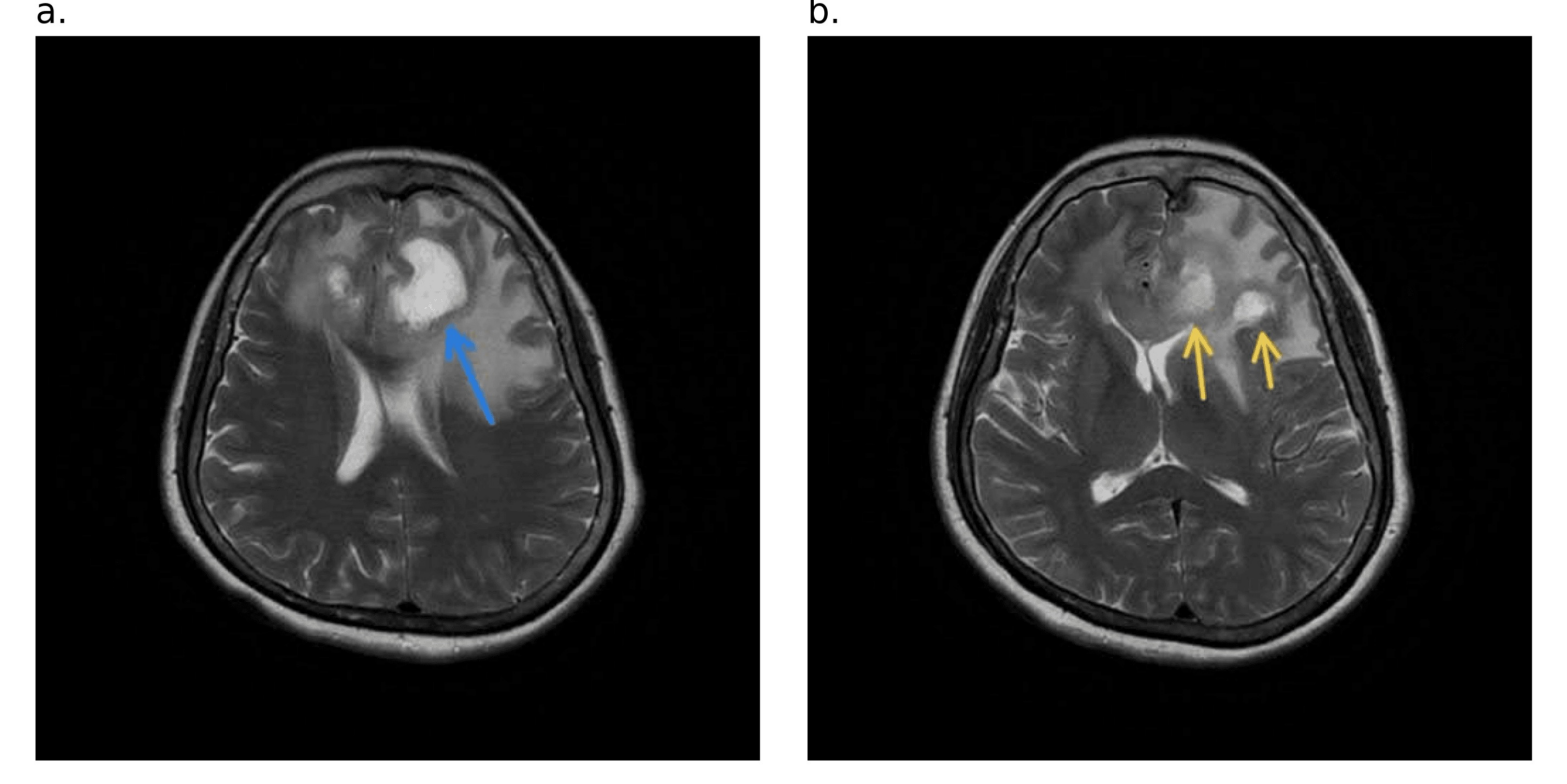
Axial T2-weighted MRI image a. Peripherally enhanced tumor (blue arrow) extending into the right frontal lobe at the level of the body of the lateral ventricles. b. Multiple peripherally enhancing tumors (yellow arrows) at the level of the capsuloganglionic region MRI: magnetic resonance imaging
